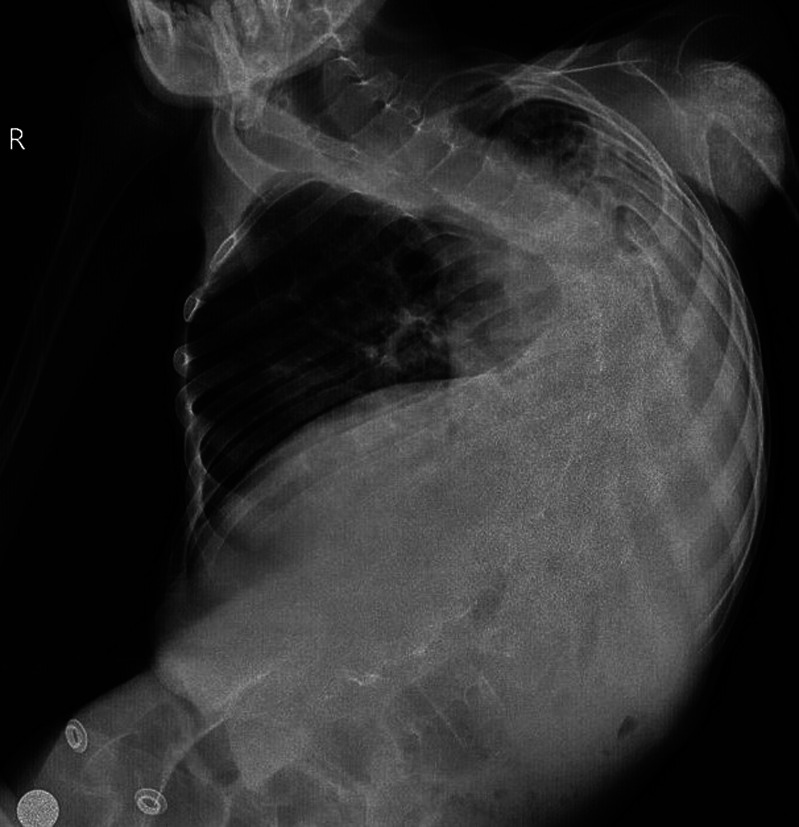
X-ray findings show serious skeletal deformities.
